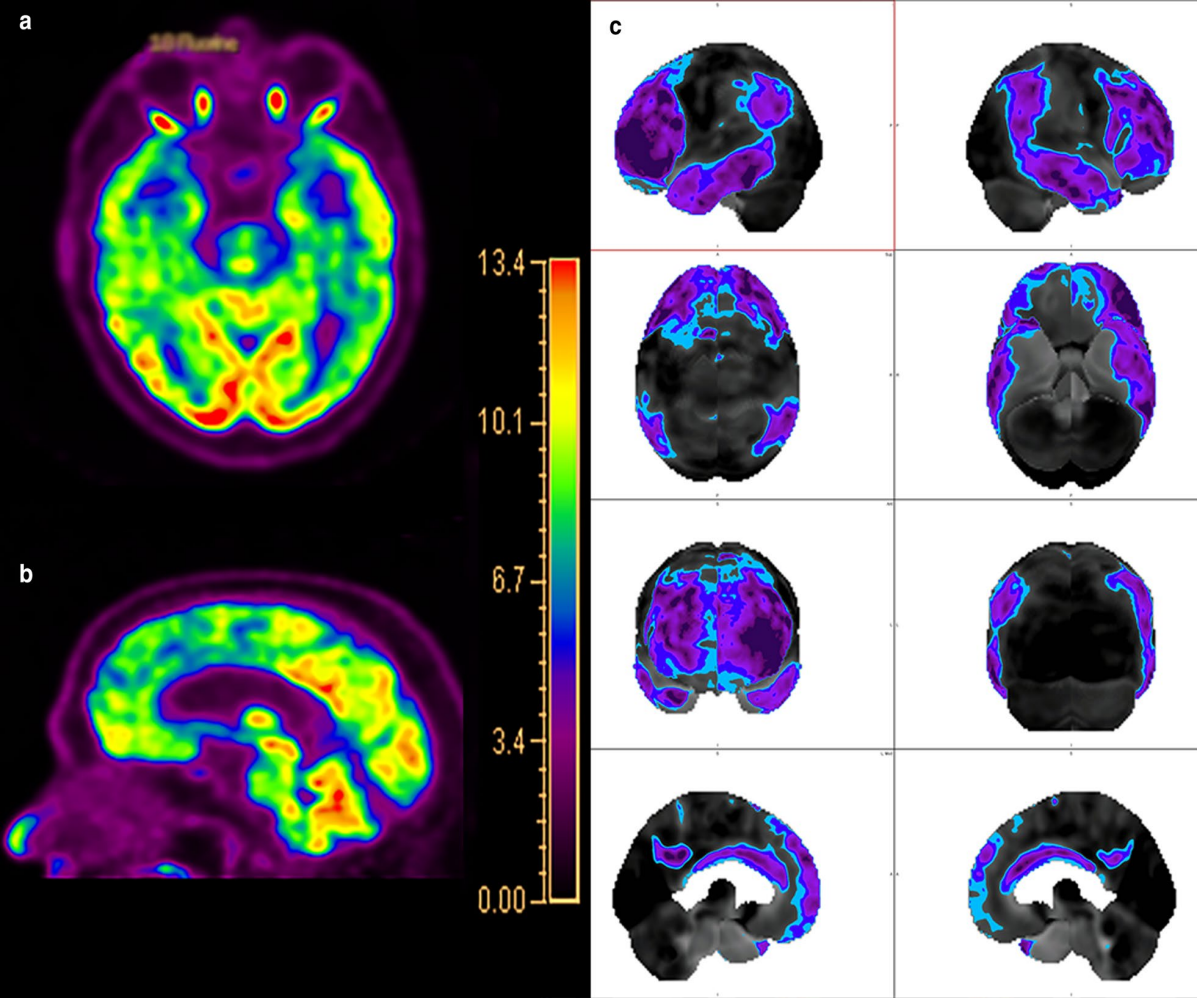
A 68-year-old man who presented to the memory care clinic with progressive personality changes and behavioral disturbances including violent outbursts and memory loss. Fluorodeoxyglucose PET/CT demonstrates marked frontal (a) and temporal (b) hypometabolism with relatively preserved parietal and occipital lobe activity including relative sparing of the PCC and precuneus. Single-subject statistical parametric mapping (c) from a similar patient demonstrates a similar pattern, with anterior greater than posterior cingulate hypometabolism, a hallmark of FTD, again with areas of blue progressing to purple representing further negative deviation from the mean uptake values of controls
